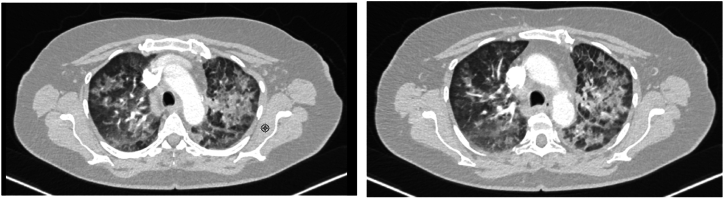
CT images of the chest: diffuse ground-glass opacities involving the entirety of both lung fields.
